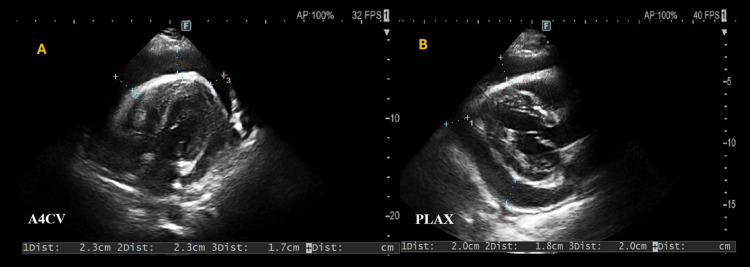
EFS measurements in the A4C and PLAX cardiac incidences in panels A and B, respectively. Panels A and B show still images acquired in the apical four-chamber (A4C) and parasternal long-axis (PLAX) views, respectively, with measurements of echo-free space (EFS) of approximately 2 cm, consistent with a large pericardial effusion.
